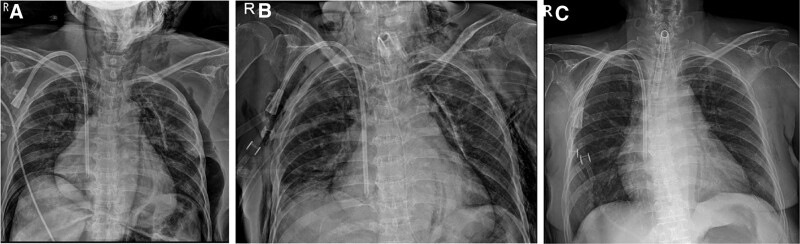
(A) Intraoperative chest X-ray. (B) Chest X-ray taken 1 day postoperatively. (C) Chest X-ray taken 3 days postoperatively.
